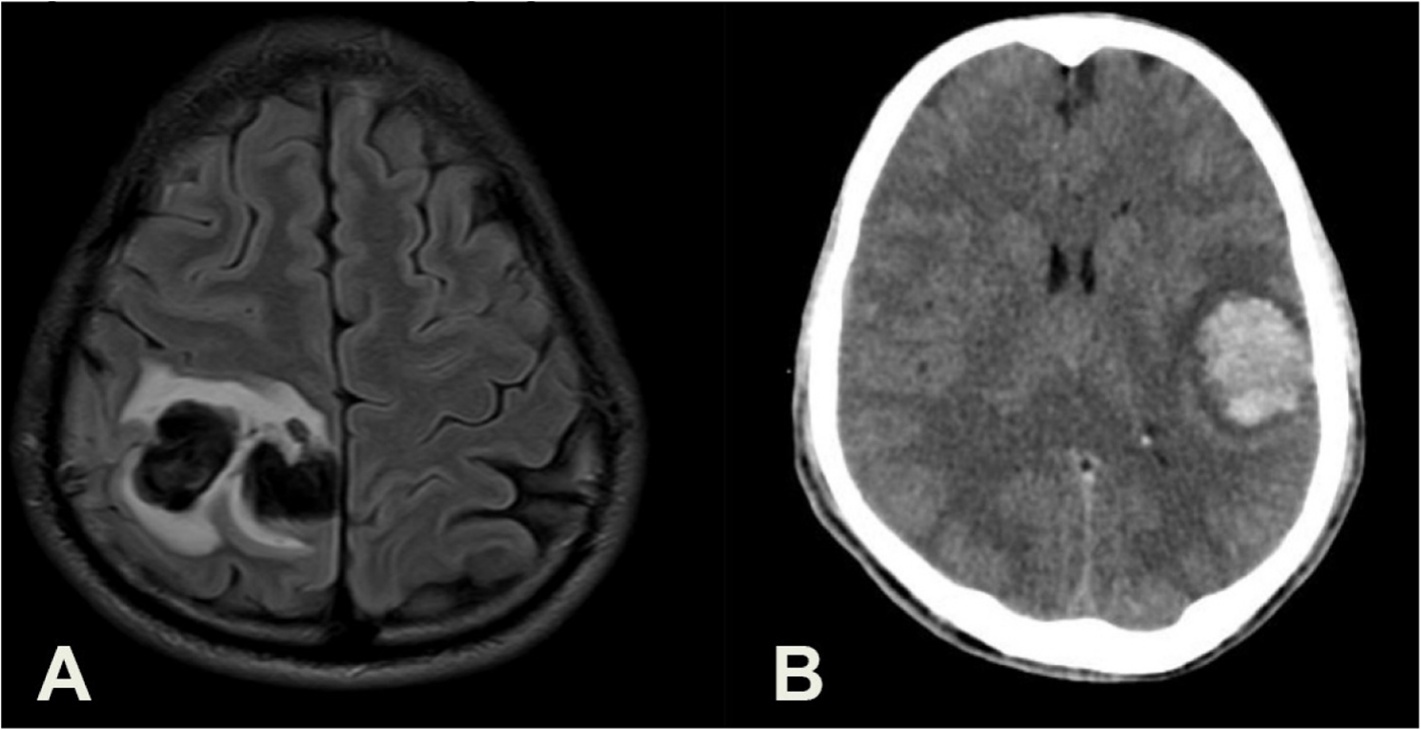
Case 1 Neuroimaging. A: Axial FLAIR MR image -right high frontoparietal hematoma (4.7 × 2.3 cm) with oedema. B: Plain CT image - worsening with a new hematoma in the left parietal lobe (4.3 × 3.3 cm)
